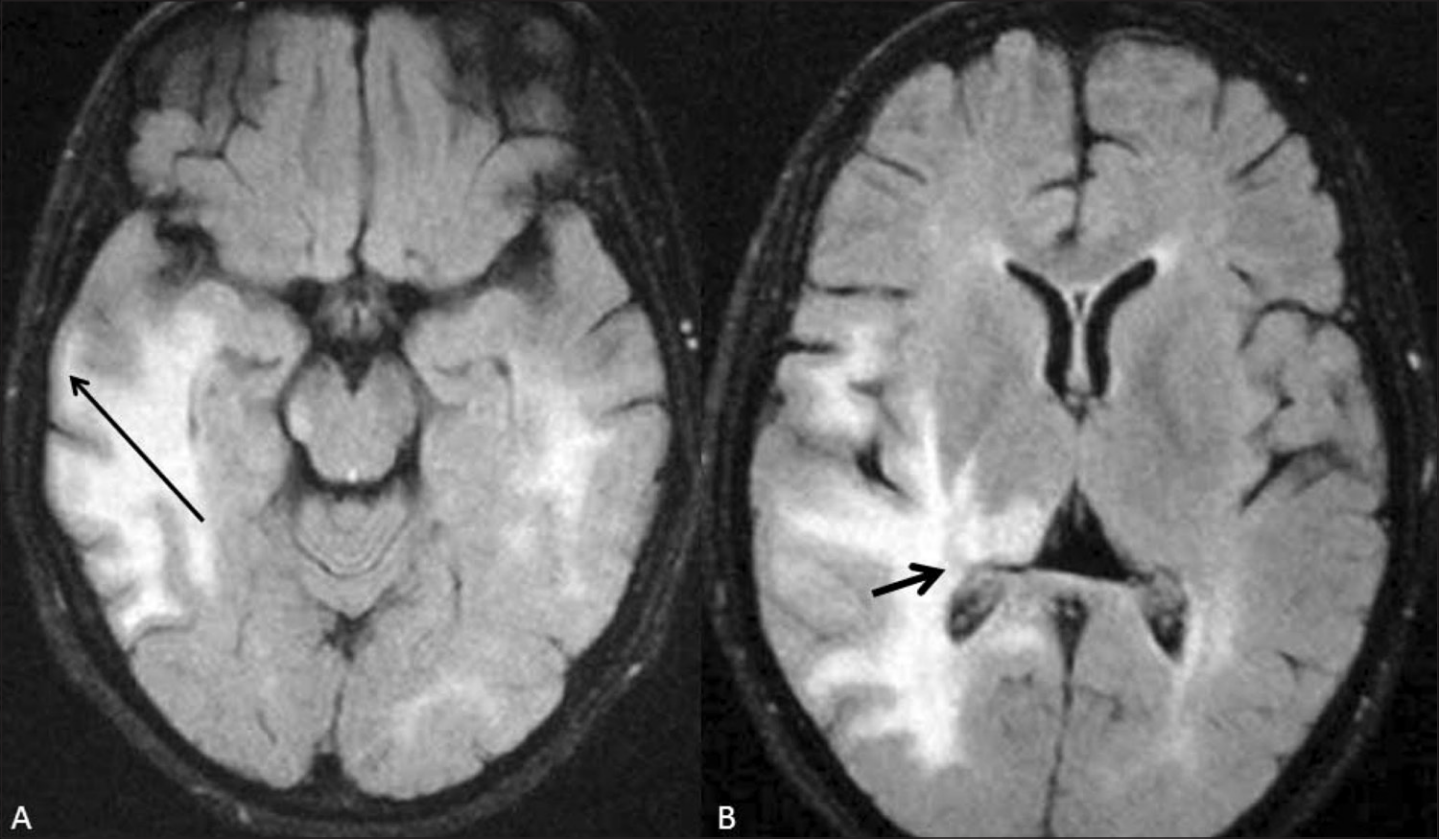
(A and B) Axial FLAIR MRI images of the brain show the T2- hyperintense lesions, seen in Figure 3, more conspicuously, especially in the subcortical (long arrow in A) and periventricular (short arrow in B) regions
